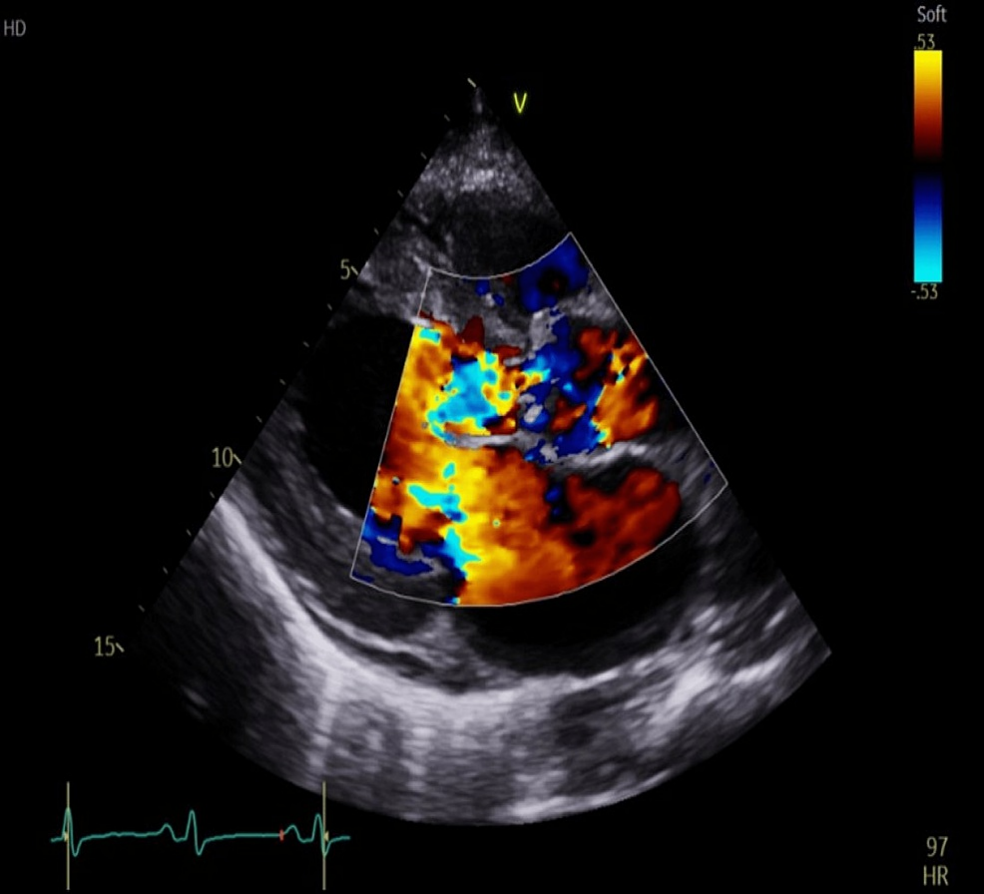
Parasternal long axis ECHO with colour flow showing severe aortic regurgitation
